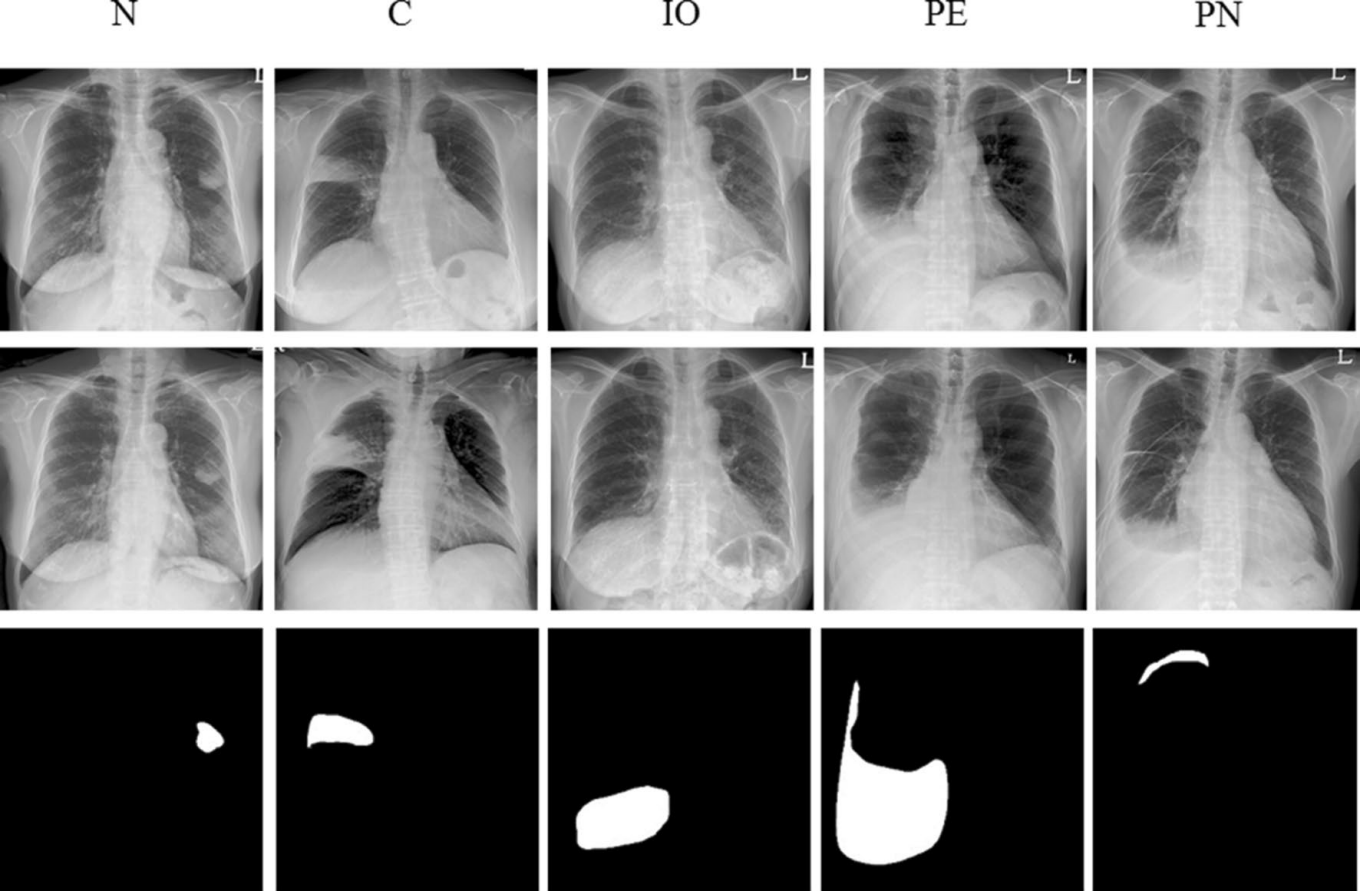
Representative examples of initial chest radiographs (CXRs) taken from each patient within a short-term interval (up rows), follow-up images of CXRs taken from each patient within a short-term interval (middle rows), and gold-standards labeled by two thoracic radiologists (bottom rows). *N* nodule, *C* consolidation, *IO* interstitial opacity, *PLE* pleural effusion, *PN* pneumothorax in Fig. 2.
